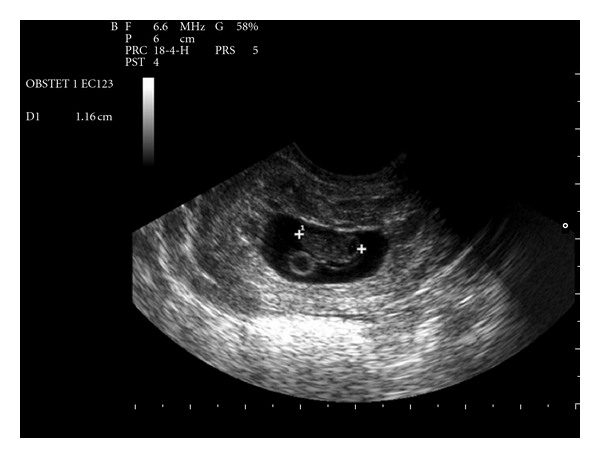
Transvaginal ultrasound image of the scar pregnancy crown-rump length 11.6 mm with fetal cardiac activity.
